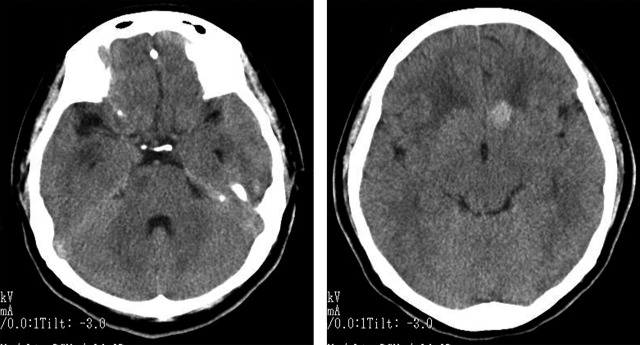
Head computed tomography images on intensive care unit admission of a 42‐year‐old Peruvian woman with fever and severe headaches, showing multiple micronodular lesions in the brain parenchyma.
